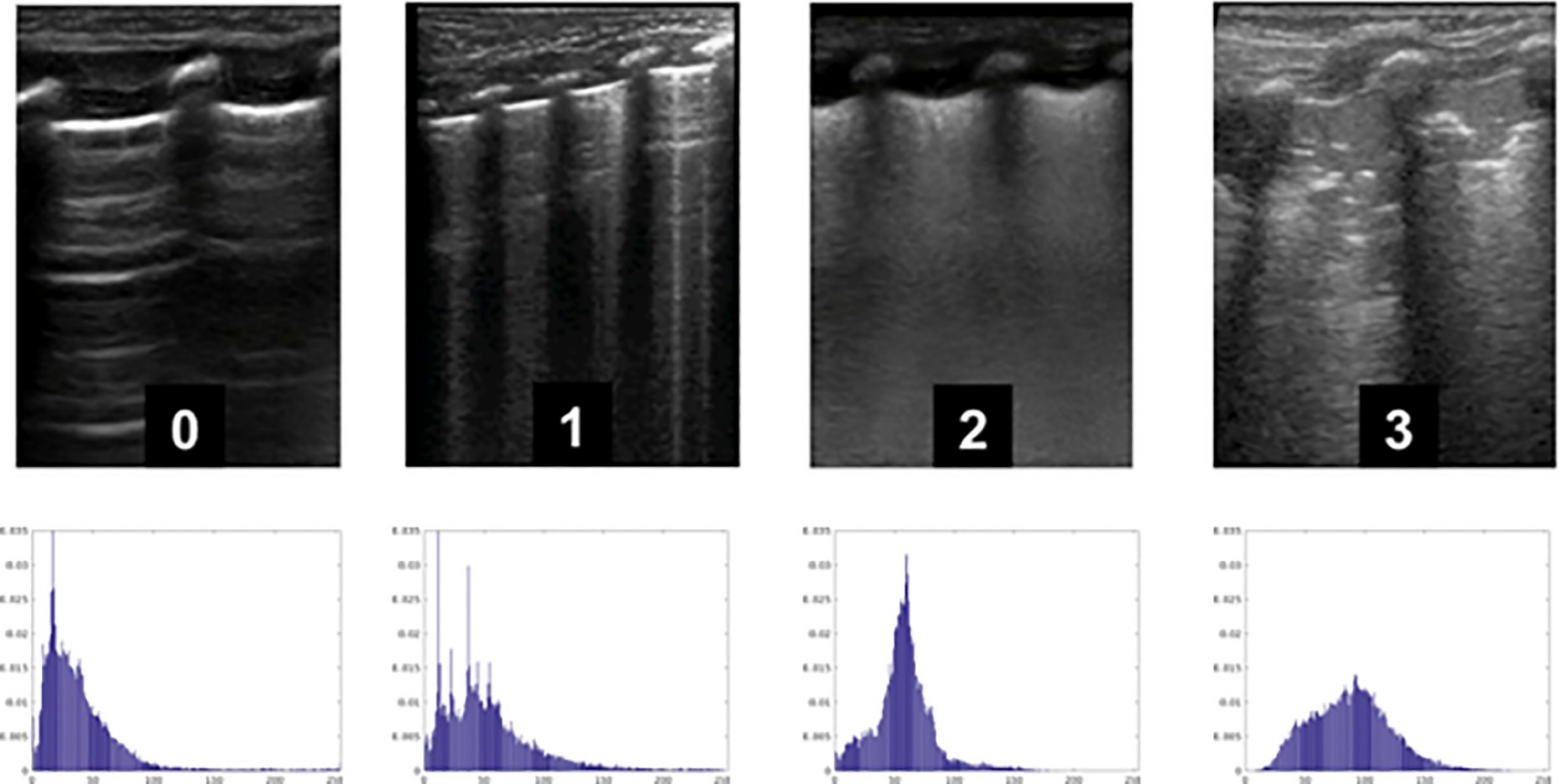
Representative lung ultrasound images used for scoring. 0 Normal pattern with horizontal reverberation of the pleural line (also known as A lines). 1 Vertical hyperechoic artifacts (also known as B lines) more than 3 per field, well spaced. Thin, regular pleural image. 2 Coalescent B lines, thick pleural image with or without small subpleural consolidations. 3 Thick and irregular pleural image with evident subpleural consolidations1.
